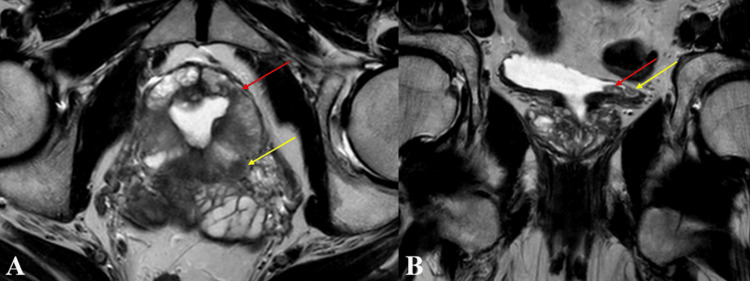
A: PI-RADS 4 lesions and distorted and dilated prostatic urethra secondary to prior transurethral resection of the prostate detected on multiparametric prostate MRI (red arrow: 7.5 mm lesion in the posterolateral left peripheral zone at the midgland level, yellow arrow: 3.5 mm lesion in the anterior peripheral zone). B: Bladder tumor detected on multiparametric prostate MRI (red arrow: 10x5 mm lesion, yellow arrow: 6x3.5 mm lesion).
